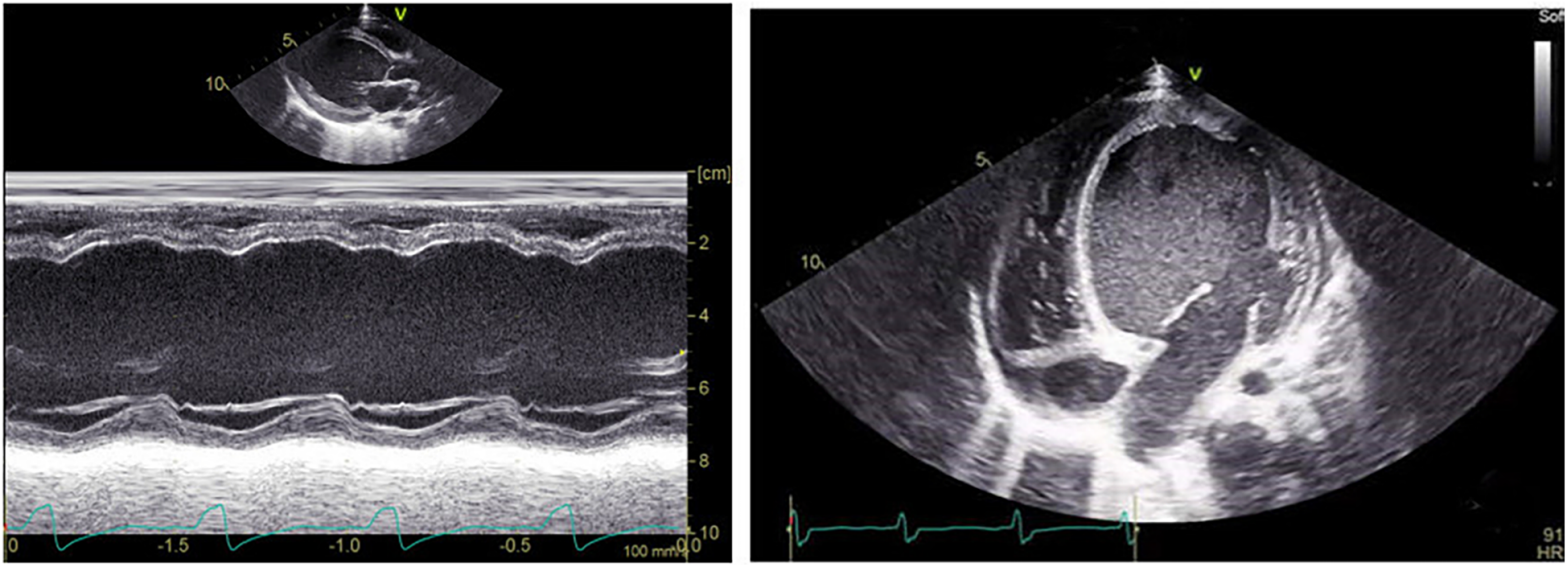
Patient, male, 11 years old, with preexcited dilated cardiomyopathy, left M-mode echocardiogram shows decreased septal and posterior left ventricular wall motion and reduced ejection fraction; transthoracic apical 4-chamber view shows spherical enlargement of the LV and thinning of the ventricular wall.
